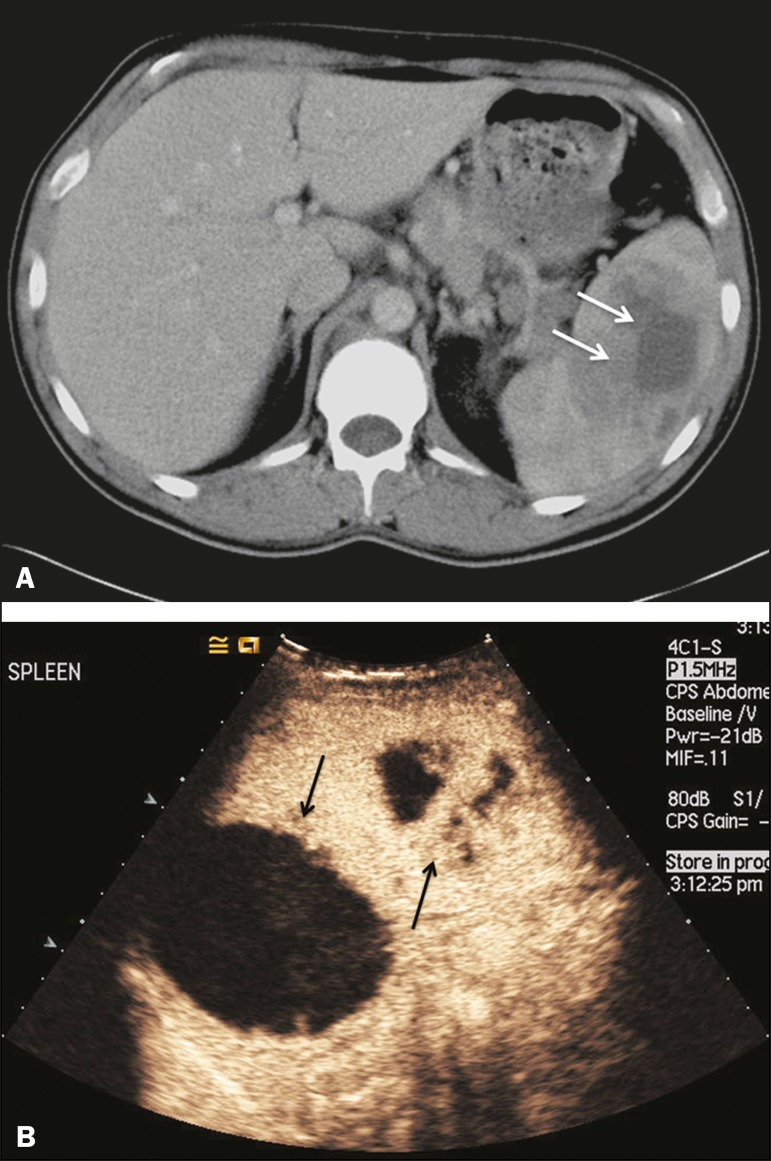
Abscess. A: Contrast-enhanced CT scan showing a
